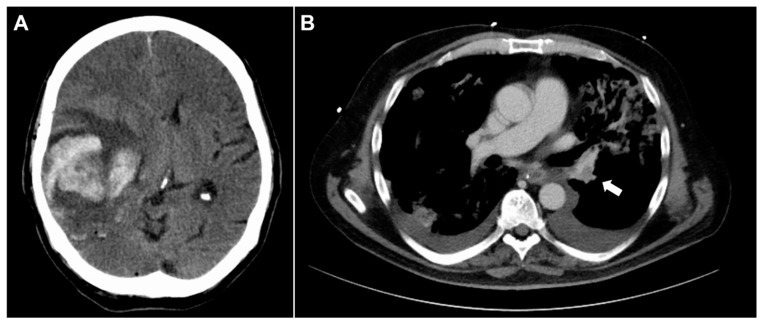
(A) Computed tomography (CT) scan of a postoperative rebleeding after glioma resection. (B) Pulmonary embolism is a complication commonly seen in neurosurgical patients.
